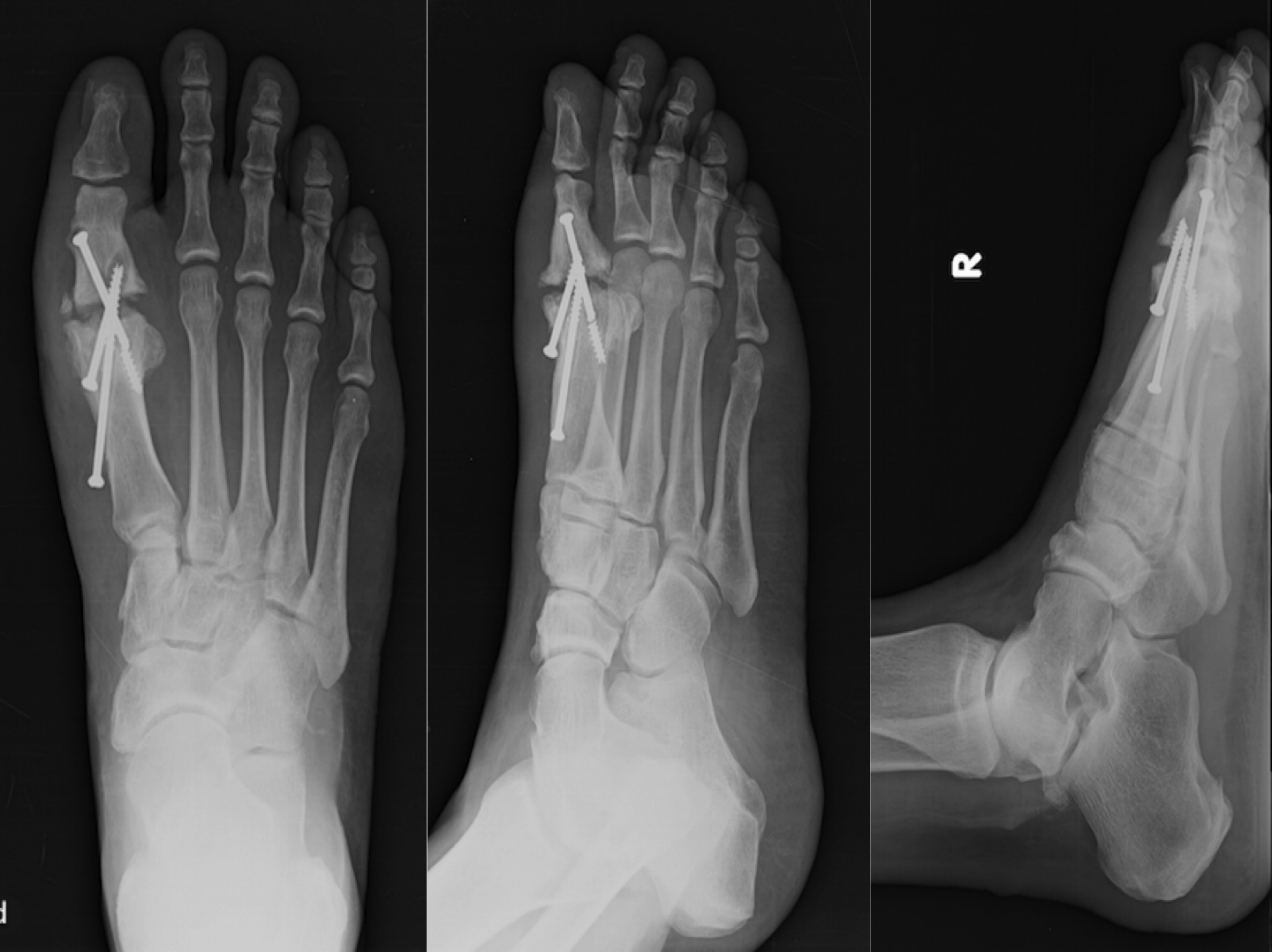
Radiographs one year post arthrodesis AP, oblique, and lateral radiographs of the patient's right foot status post MTP arthrodesis with lucencies surrounding the cannulated screws as well as a broken screw demonstrating a non-union of the right first MTP joint.
